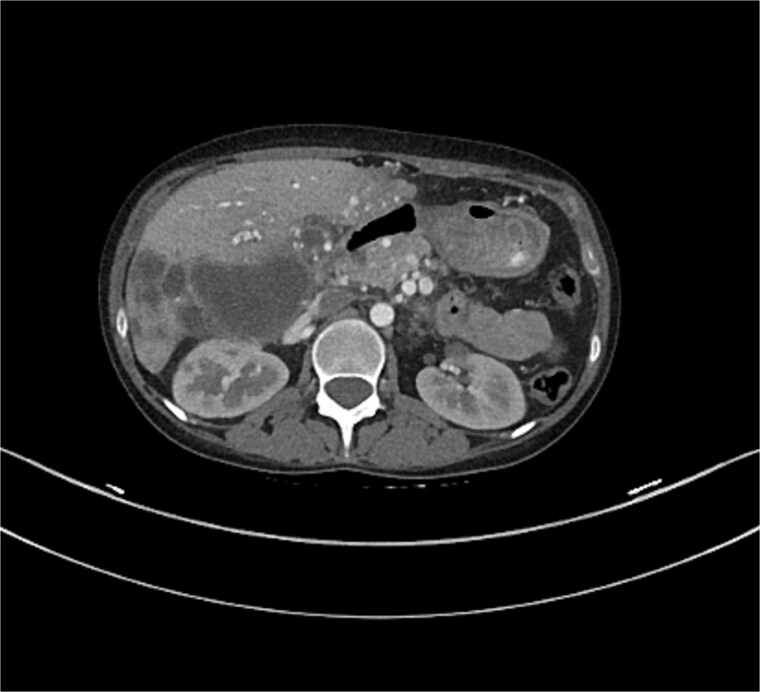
CT of the liver post-receiving chemotherapy by three months.
